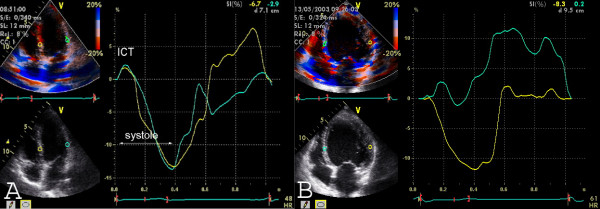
Assessment of asynchrony by strain from the apical four chamber view. The sample volumes are in the basal septal and the basal lateral segments. A) Normal strain curve in a control patient. ICT = isovolumetric contraction time. B) Strain curve with asynchronous myocardial velocity in the septal (=yellow) and the lateral (=green curve) segment.
